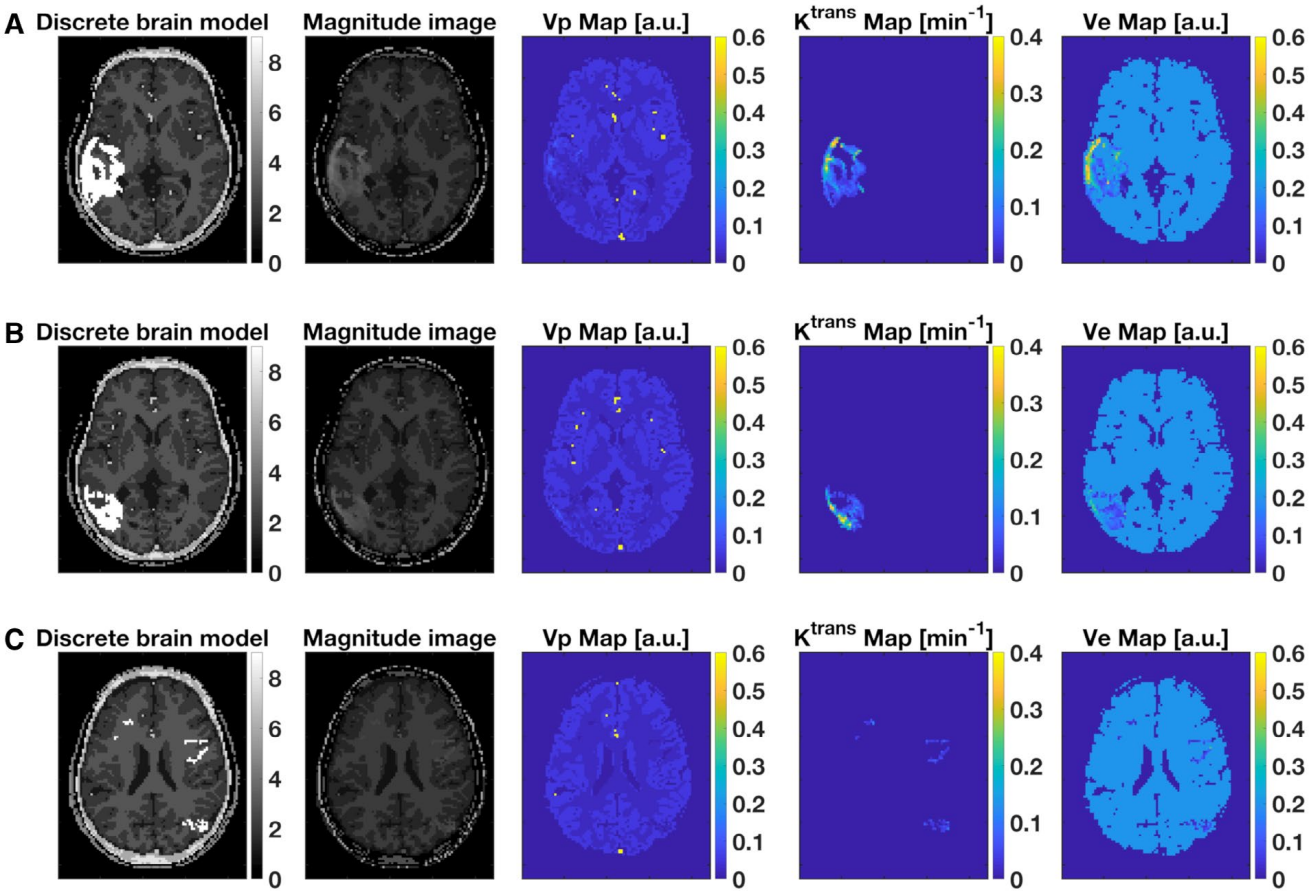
Three DROs were used to test for robustness of the results: Brain slice with large tumor with enhancing rim (A), brain slice with smaller tumor (B), and brain slice with metastases (C). These were formed by inserting previously developed brain tumor DCE‐MRI reference objects^38^ into brain data from subject 4 of the BrainWeb Database.^68^ Through this process, additional DROs can be easily created
